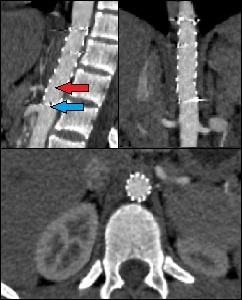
Follow-up computed tomography angiography 2 months after endovascular repair of the aortic pseudoaneurysm. It is possible to observe the wound has sealed and the celiac trunk is covered by the endoprosthesis, with flow maintained inside it from collaterals (red arrow). Note that the superior mesenteric artery is patent (blue arrow).
